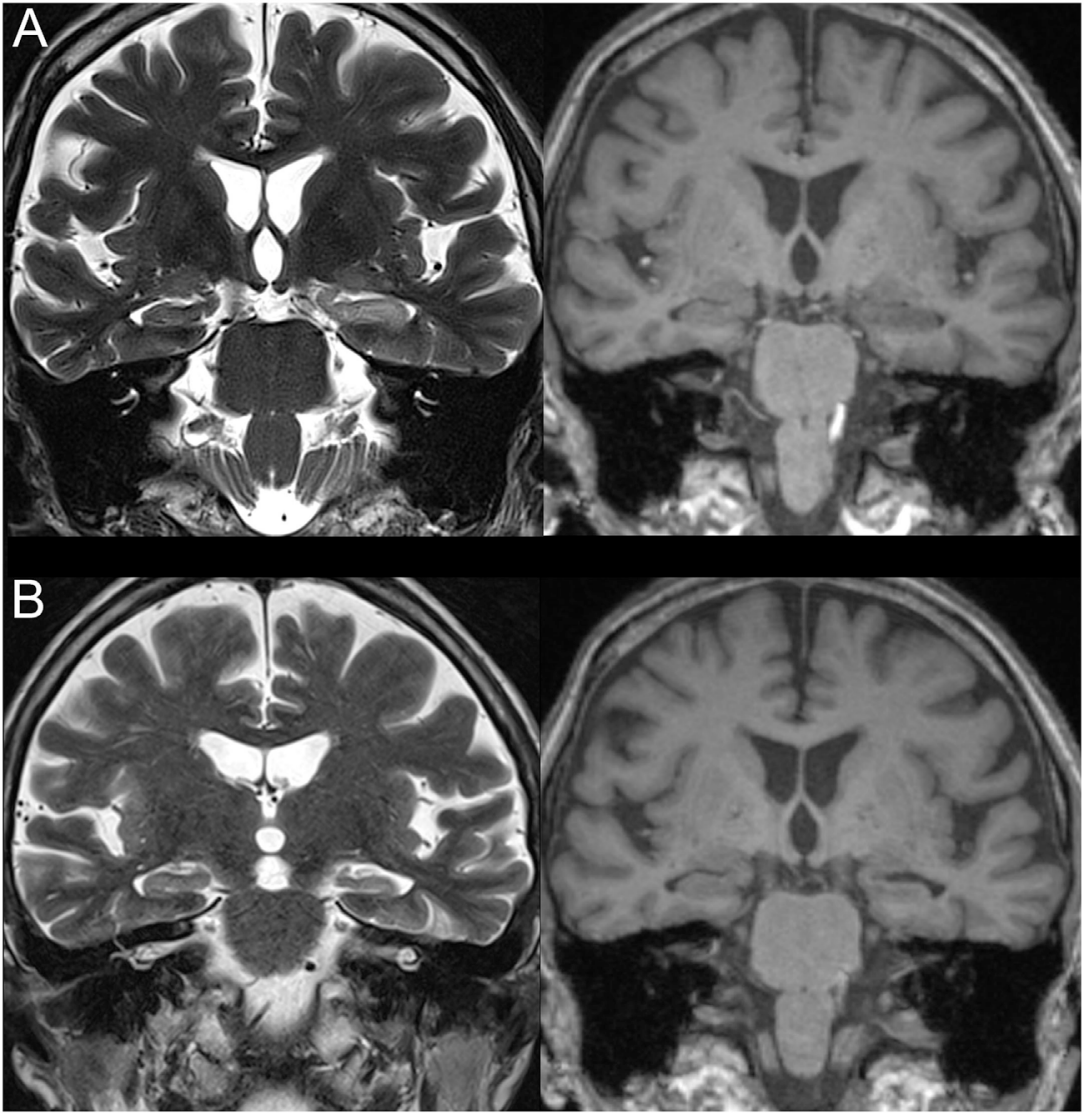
T1- (right) and T2- (left) weighted magnetic resonance imaging images (coronal slices). (A) Increased left hippocampal volume with increased T2 and decreased T1 signals. (B) Reduced left hippocampal volume without signal changes.
